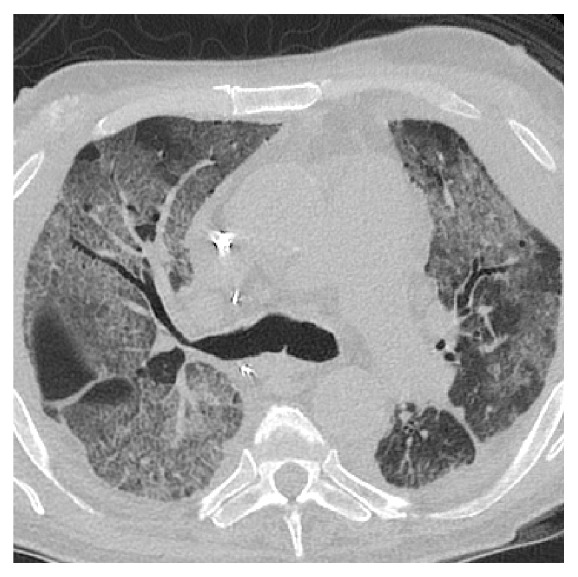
Transverse chest computed tomography demonstrating widespread peribronchovascular ground-glass opacities throughout both lung fields.
